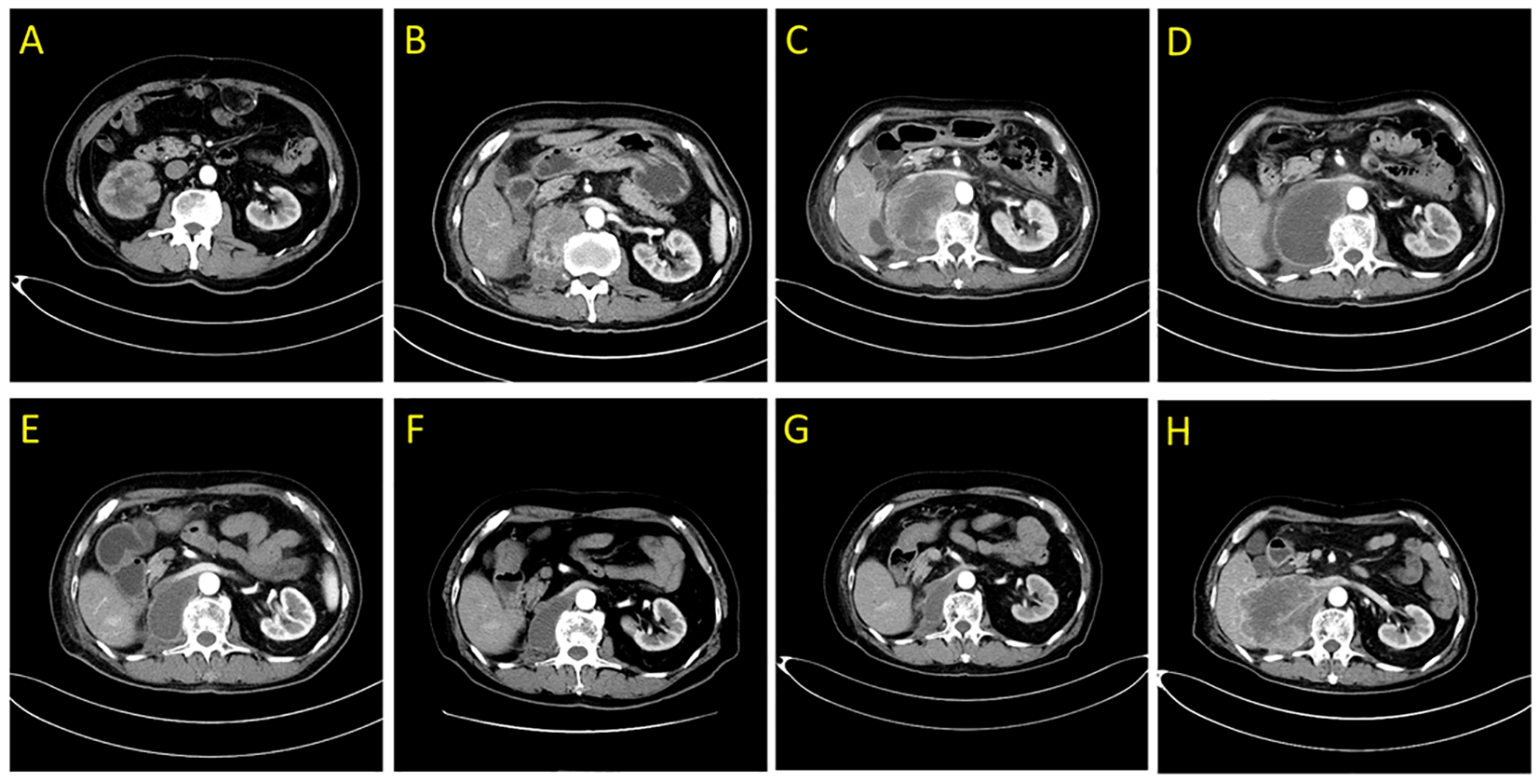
Abdominal enhanced computed tomography scans demonstrating the right posterior peritoneal tumor lesion. (A) at diagnosis in February 2020; (B) at disease relapse 4.5 months after RNU; (C) the tumor lesion was enlarged following 2 weeks of first dose of tislelizumab treatment in July 2020; (D) liquefaction and cystic changes were observed within the tumor lesion following 6 weeks of tislelizumab combined with anlotinib treatment in August 2020; (E–G) continuous shrinkage of the tumor lesion following tislelizumab combined with anlotinib treatment in October 2020, January 2021, and May 2021, respectively; (H) disease progression following 20 months of tislelizumab combined with anlotinib treatment in February 2022.
